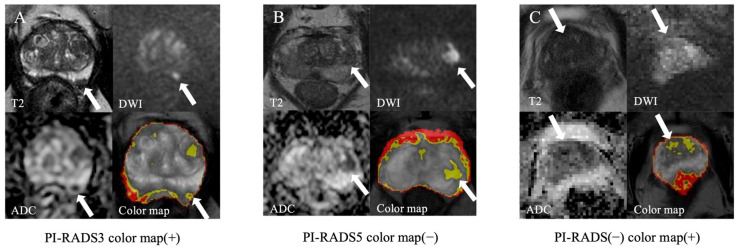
Typical examples of color maps by the PI-RADS score. (A) A small lesion in the left peripheral zone, which appeared hypointense on the axial T2-weighted image, was hyper- and hypointense on diffusion-weighted imaging and the apparent diffusion coefficient map, respectively, and was assigned a PI-RADS 3. This lesion was positive on the color map. (B) A large lesion in the left peripheral zone, which appeared hypointense on the axial T2-weighted image, being hyper- and hypointense on diffusion-weighted imaging and the apparent diffusion coefficient map, respectively, was assigned a PI-RADS 5. However, this lesion was negative on the color map and biopsy also yielded negative results for clinically significant prostate cancer. (C) This case highlights a color map-positive lesion, which was not suspicious for clinically significant cancer pre-biopsy MRI.
